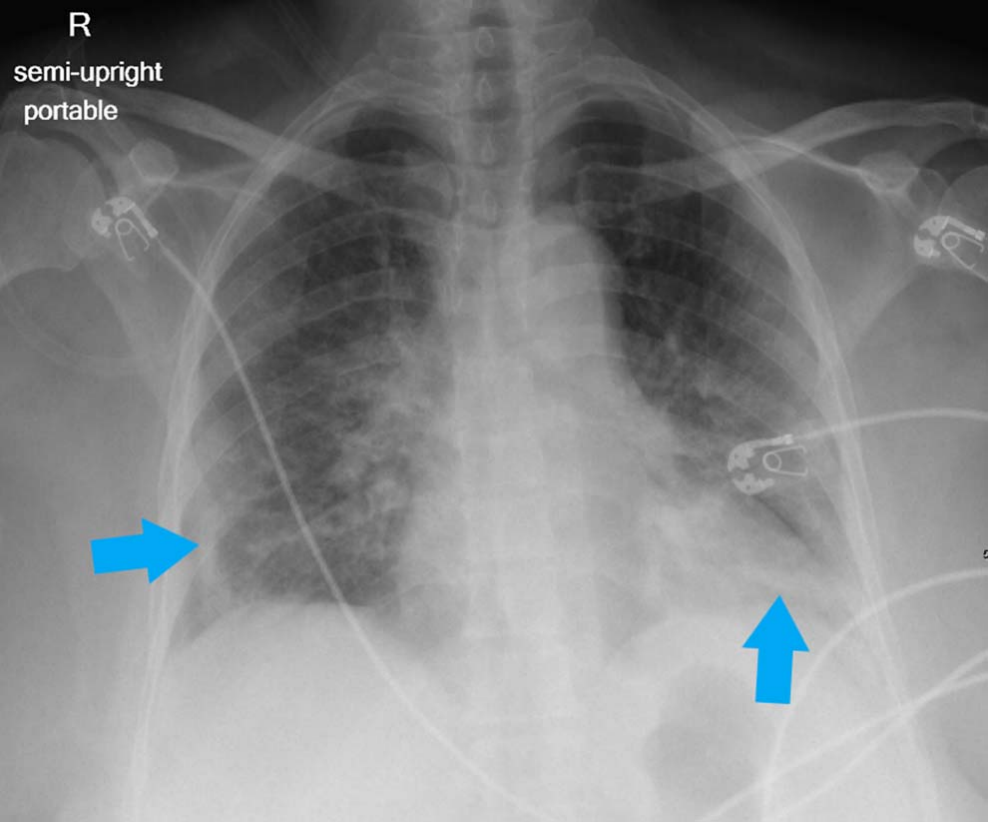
Portable semi-upright anteroposterior radiograph of a 66-year-old female shows characteristic bilateral confluent, bandlike (arrows) consolidative opacity in a peripheral, mid-to-lower lung zone distribution admixed with patchy airspace opacity, greatest at the left lung base.
